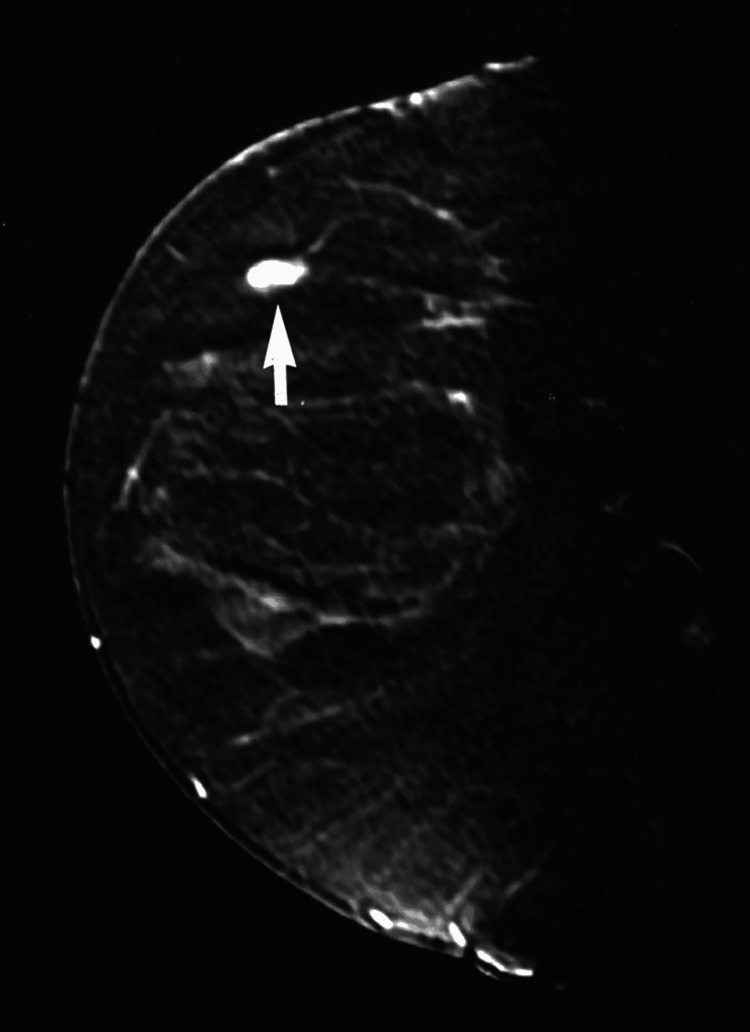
Sagittal T1-weighted post-contrast MRI of the left breast Sagittal MRI of the left breast in a 19-year-old female shows a small, well-circumscribed, oval lesion within the upper outer quadrant (arrow) measuring approximately 2 cm. The lesion demonstrates homogeneous signal intensity without spiculation, abnormal enhancement, or axillary adenopathy, consistent with a benign BI-RADS 3 finding in a post-radiation survivor.
